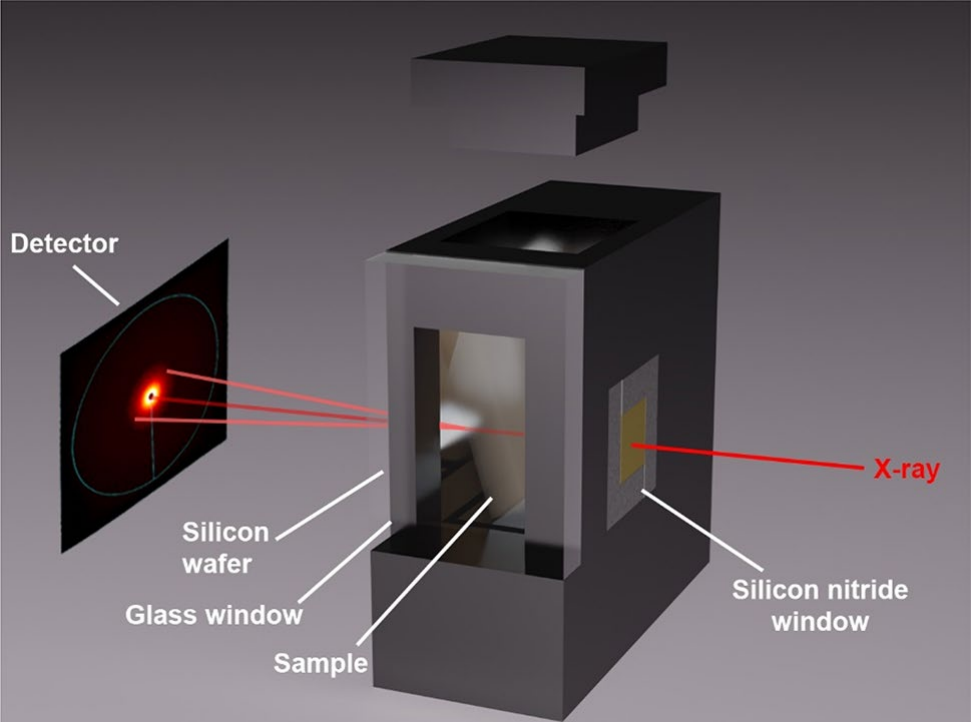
Rendering (Blender v2.90.1 https://www.blender.org/) of the in-situ measurement cell and the x-ray pathway through it. The measurement cell consists of anodized aluminium and is sealed with a silicon wafer, a silicon nitride window, a museum-glass window and an aluminium lid.
